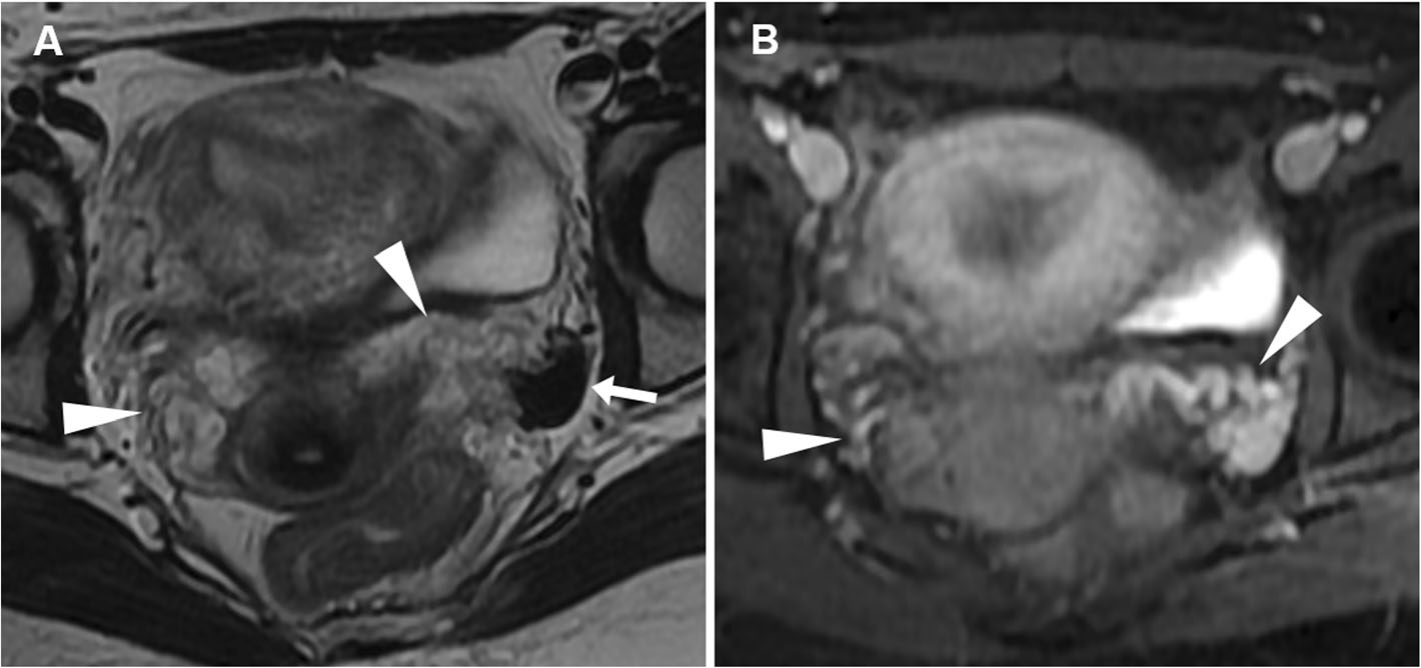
MRI of pelvic congestion syndrome in a 26-year-old woman with chronic pelvic pain and a recent severe exacerbation. Oblique-coronal T2-weighted image (a) shows ectatic vascular structures in the parametria, showing predominantly high signal intensity (arrowheads); in the left parametrium, some varices (arrow) display low signal intensity. Corresponding oblique-coronal gadolinium-enhanced fat-suppressed T1-weighted image (b) demonstrates vascular enhancement of the abovementioned structures (arrowheads), thus confirming pelvic varices
